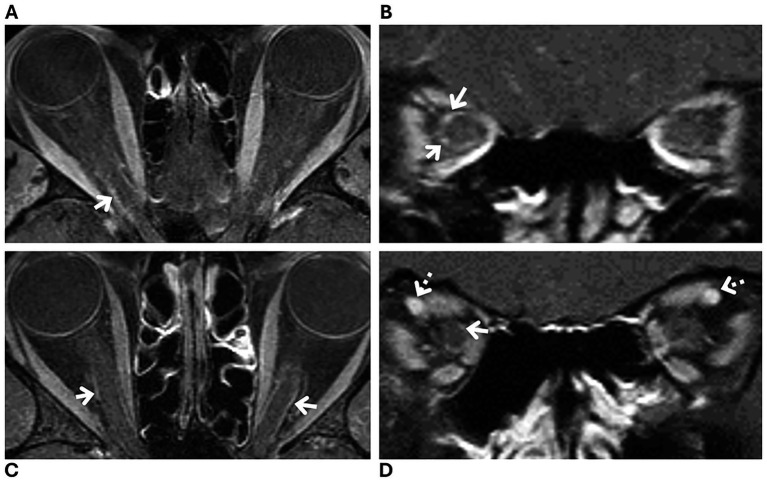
Magnetic resonance imaging (MRI) of two patients with a new diagnosis of idiopathic intracranial hypertension, showing prominent blood vessels within the optic nerve sheath (white arrows) without definite enhancement. Fat-suppressed T1-weighted orbital MRI with contrast (A–D) in axial (A,C) and coronal (B,D) planes: (A,B) from one patient and (C,D) from another. Blood vessels (white arrows) that mimic optic nerve sheath enhancement on axial planes (A,C) are more clearly identified as vessels on coronal planes (B,D). Note the prominent ophthalmic vein on the coronal plane in one patient, suggestive of venous congestion (dashed arrows, D).
